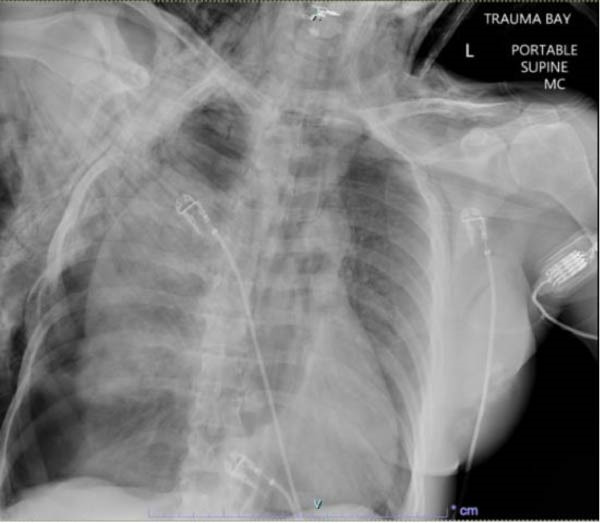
Initial chest radiography on arrival in trauma bay. A radiograph potentially shows the “fallen lung sign,” a rare but specific indication of tracheobronchial injury in the case of persistent pneumothorax.
